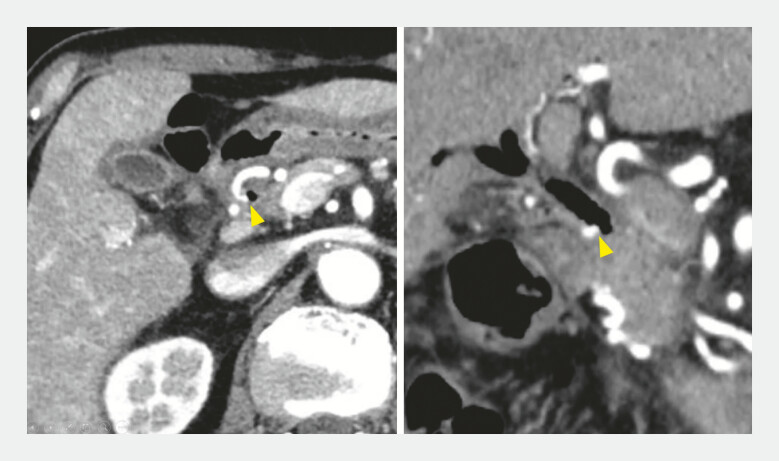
Contrast-enhanced computed tomography scan showing the displaced fully covered self-expandable metal stent in the bowel and the right hepatic artery pseudoaneurysm on the common bile duct wall (arrowhead).
